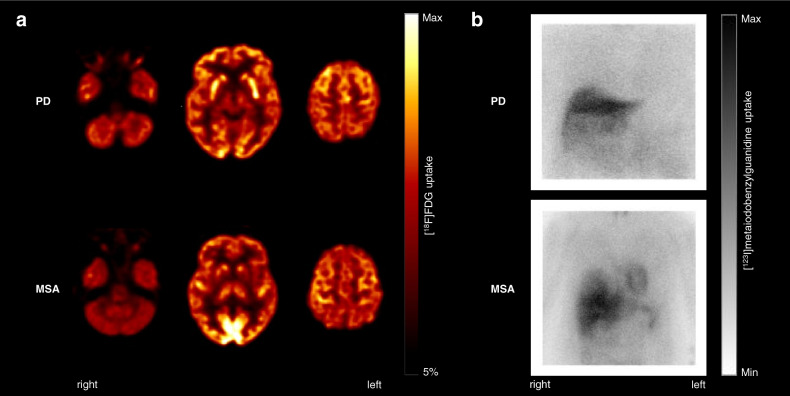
Typical FDG PET and MIBG scintigraphy findings in individual patients with Parkinson’s disease and multiple system atrophy. a Spatially normalized transaxial FDG PET slices at the level of cerebellum, basal ganglia, and dorsal frontoparietal cortex. Datasets were thresholded for optimal display. b Anterior view of planar MIBG scintigraphy. The patient with PD shows a typical relative hypermetabolism of the bilateral putamen and no cardiac MIBG uptake. The MSA patient is characterized by hypometabolism of the putamen (particularly on the left side), the bilateral cerebellum, and a preserved cardiac uptake on MIBG scintigraphy. *PD* Parkinson’s disease, *MSA* multiple system atrophy.
